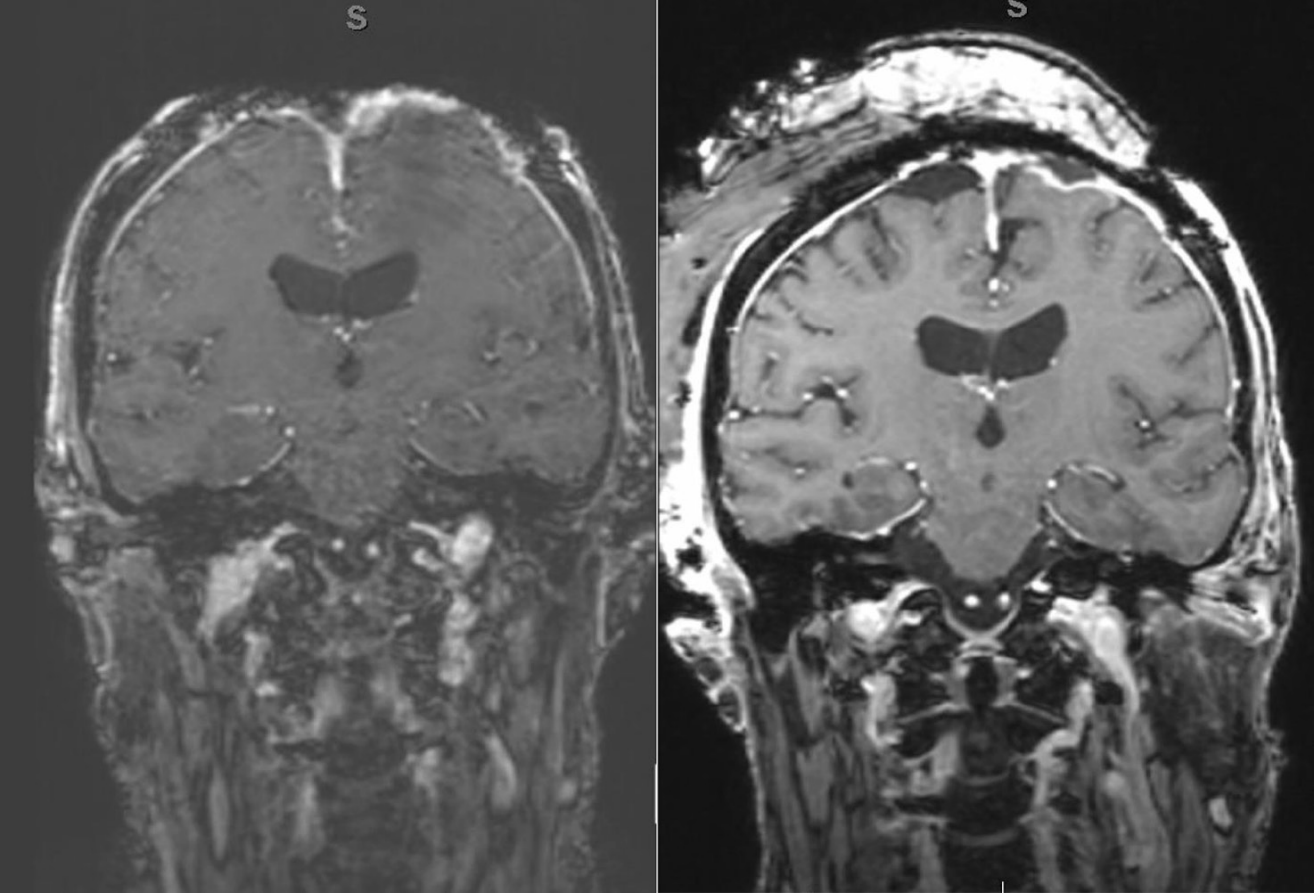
Neuroradiologic magnetic resonance imaging during acute cerebritis (Left) and similar imaging obtained 1 year prior (Right).
